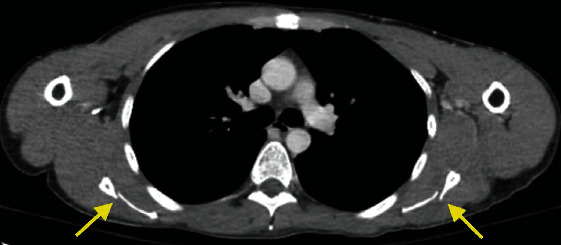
Computed tomography (CT) imaging demonstrating bilateral scapular body fractures.
